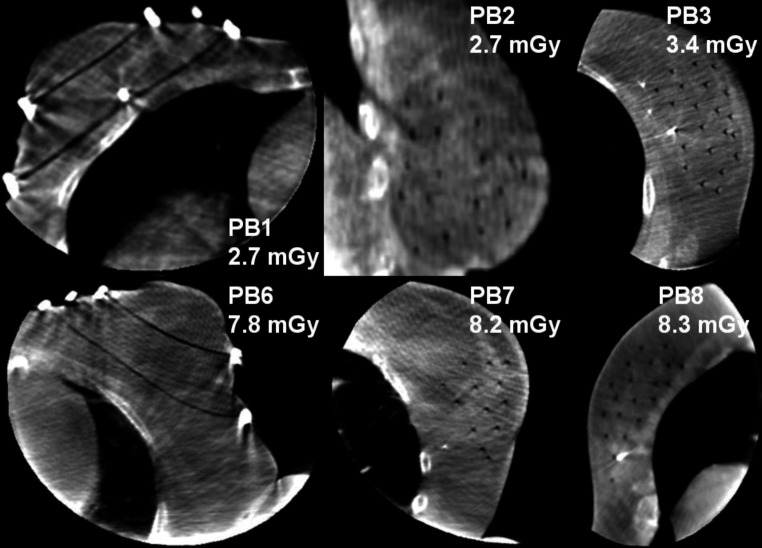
Shown are six representative control-CBCTs of breast patients. Patient IDs and CBDI_w_ as dose metric are provided. Catheters and surgical clips were distinctly identifiable in each case. Level: −40 HU, Width: 700 HU. *CBCT* cone-beam computed tomography, *Patient ID* patient identification, *CBDI*_*w*_ weighted cone-beam dose index
